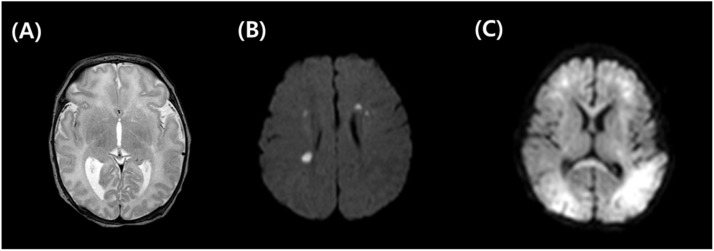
Comparison of normal vs. abnormal brain MRI. Normal brain MRI, T2 weighted (A), abnormal brain MRI with multifocal periventricular white matter lesions, diffusion weighted image (B), abnormal brain MRI with global ischemic change both subcortical and white matter, diffusion weighted image (C).
